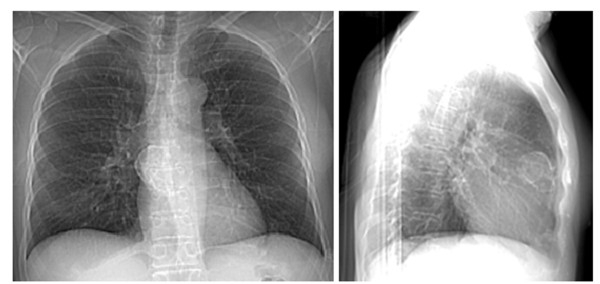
Frontal chest radiograph shows a rim calcified lesion (left panel). Lateral chest radiography confirms that the rim calcified mass resides within the anterior mediastinum (right panel).
